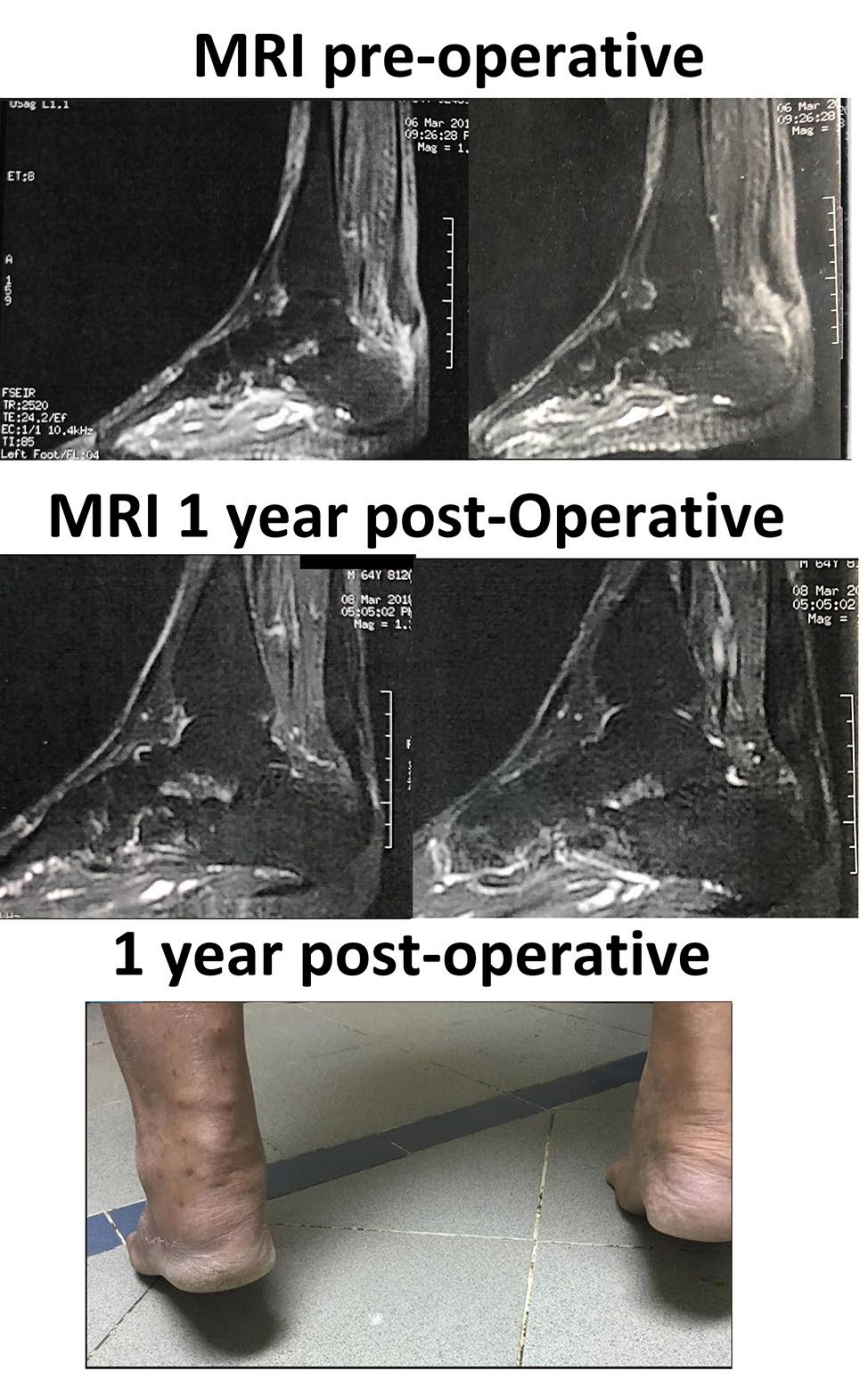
Preoperative and Follow-up MRI at one year - Clinical evaluation at one-year follow-up
